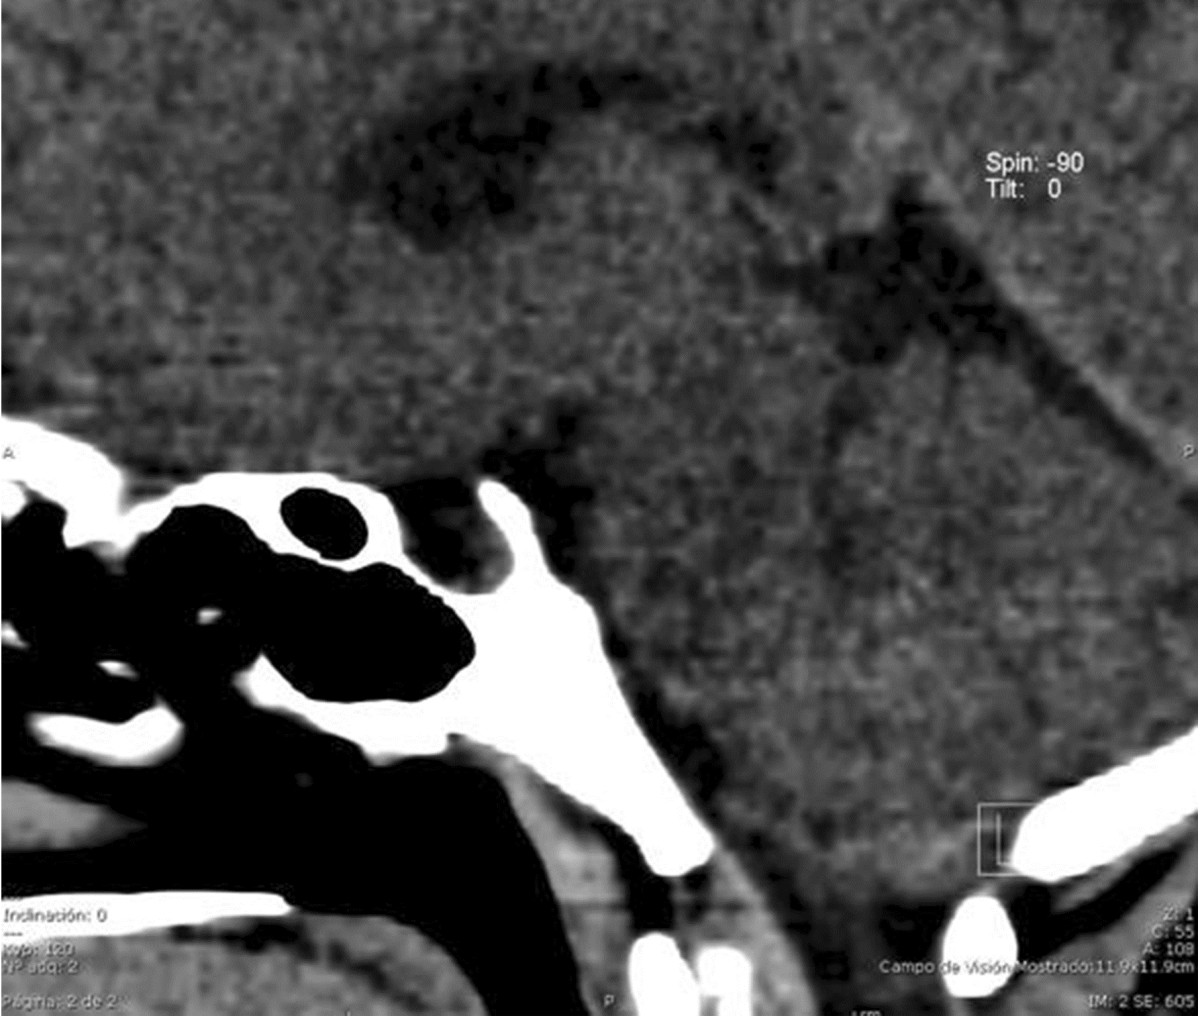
Sagittal computed tomography scan of the sella. Arachnoidocele compression of the pituitary gland in 70% is shown.
